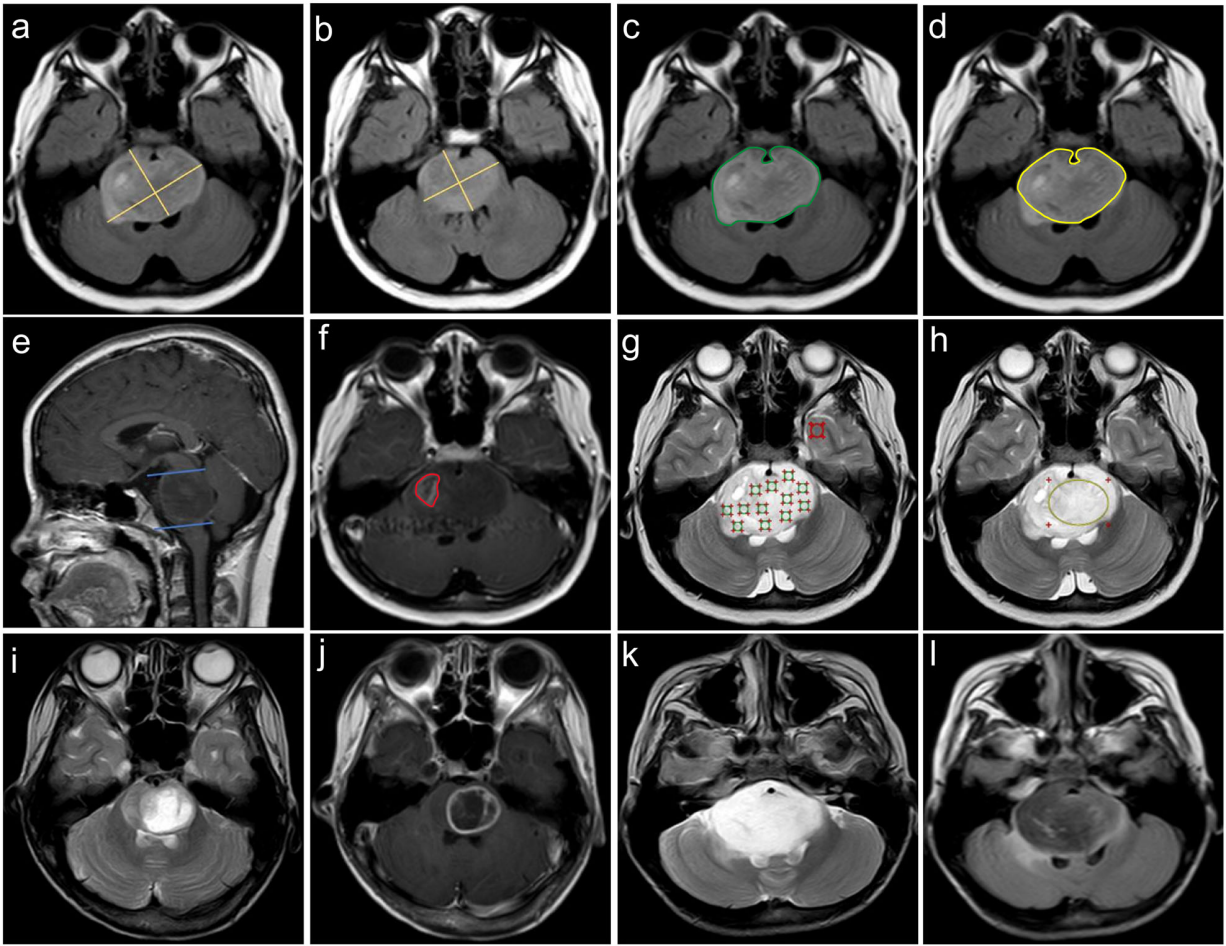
Measurement of the perpendicular diameters 2D products, T2 signal intensity, and volume (including total tumor, pons, and ring enhancement) and DIPG morphologic characteristics. a, b Measurement of 2D products of the largest perpendicular diameters before and after DIPG radiotherapy. c–f Measurement of DIPG volume: including total tumor volume (green), pontine tumor lesion volume (yellow), and ring-enhancement volume (red). g, h Minimum, and maximum T2SI ( ≥ 8 per patient; green), Mean T2SI (yellow), and normal-appearing gray matter of the temporal lobe T2SI (red). i, j An area in a tumor with clear boundaries and liquid-like signals. Contrast-enhanced T1-weighted image surrounds high signal. k, l T2-weighted hyperintense and FLAIR images showing substantially lower signal. DIPG, diffuse intrinsic pontine glioma; T2SI, T2 signal intensity
